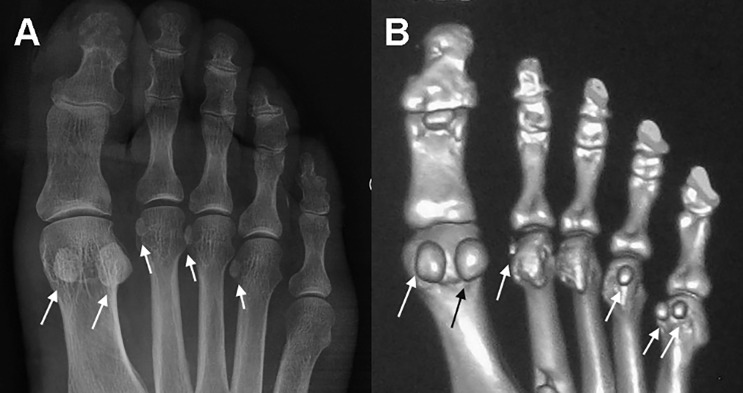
Anteroposteriorradiographs (A) and three dimensional reconstruction computed tomography scans (B) of the forefoot of two adults, with the location of sesamoid bones at the metatarsophalangeal joints indicated by arrows.
